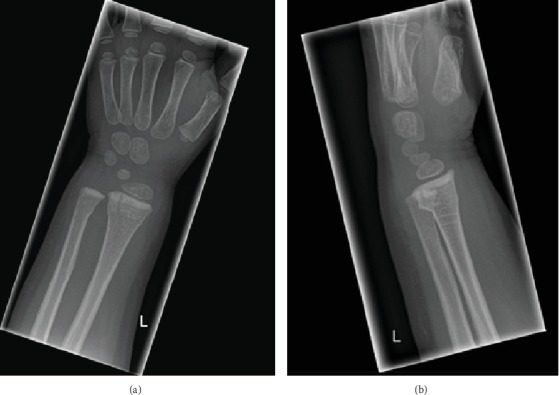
(a, b) Anteroposterior and lateral radiographs of the left wrist showing Salter-Harris type 2 fracture of the distal radius, with minimal dorsal displacement and a small line on the ulna suggestive for an ulnar fissure.
